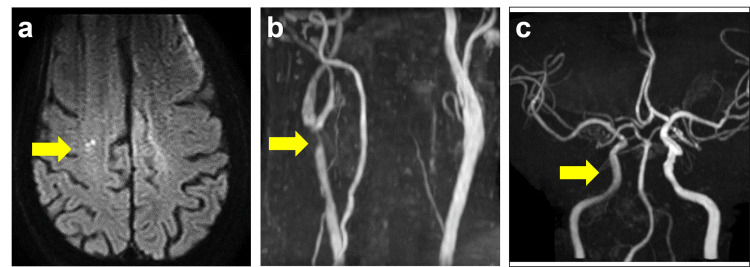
Preoperative images a) Head MRI diffusion-weighted image shows an acute cerebral infarction in the right frontal subcortical white matter (arrow). b) Cervical MRA demonstrates severe stenosis of the right intracranial carotid artery (ICA) (arrow). c) Head MRA shows poor visualization of the right ICA and middle cerebral arteries (arrow).
